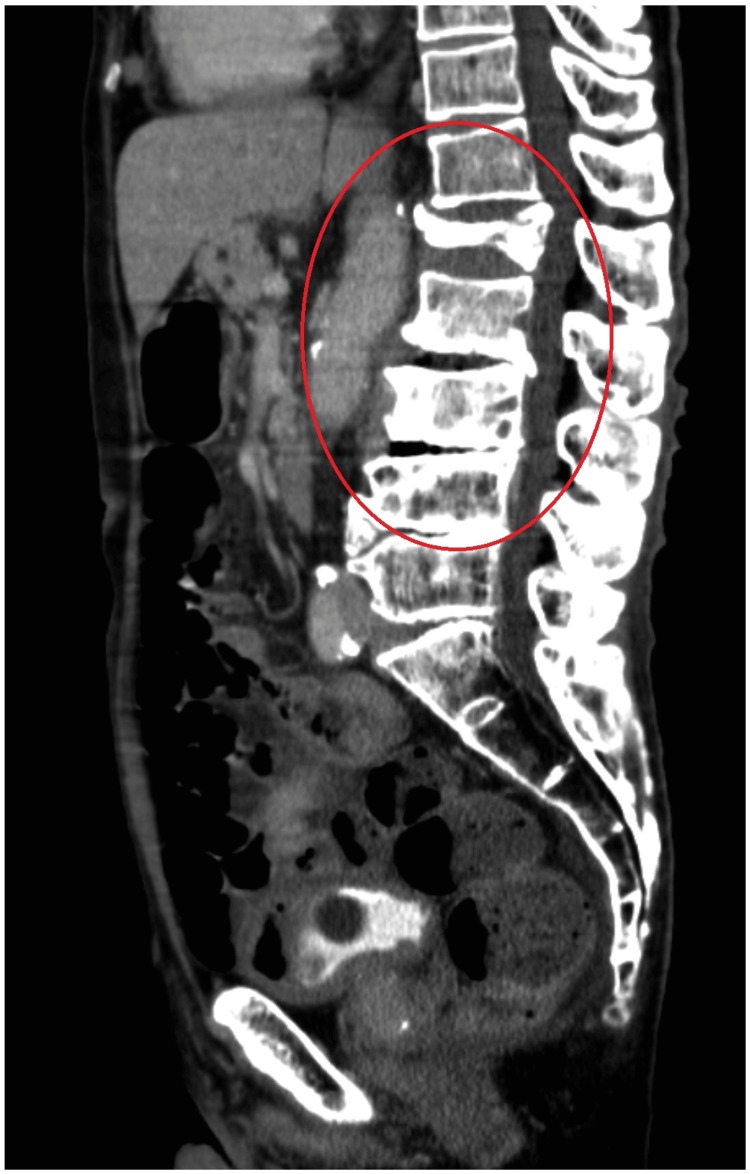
Computed tomography image of the lumbar spine Computed tomography image of the lumbar spine showing gas production in the L2/3 and L3/4 discs (red circle)
